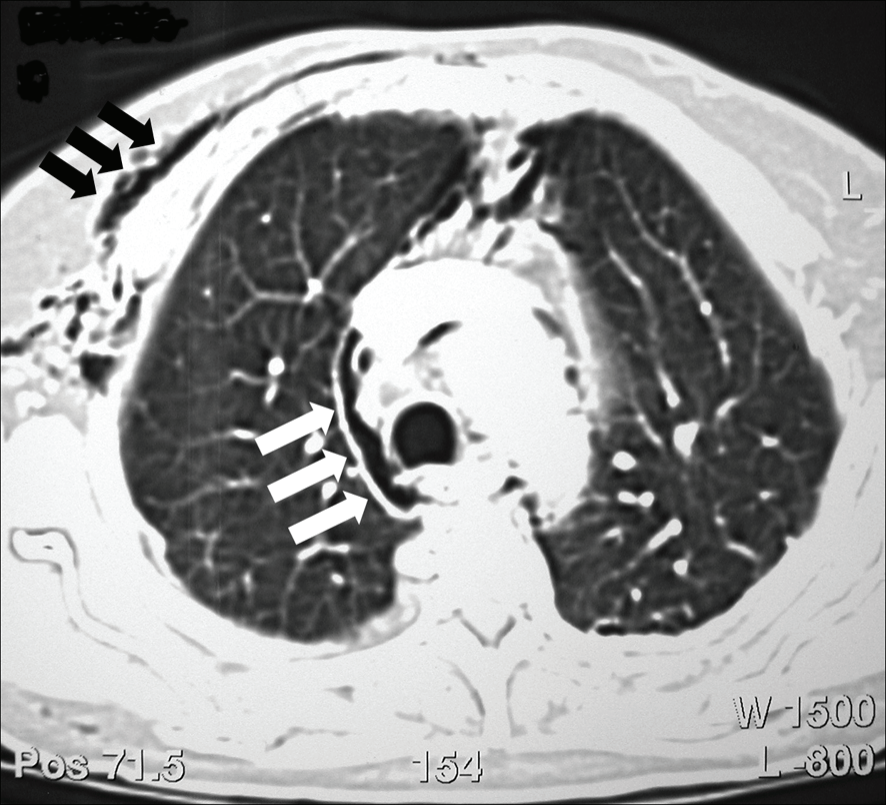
Thoracic computed tomography (CT) showing pneumomediastinum (white arrows) and subcutaneous emphysema (black arrows).
